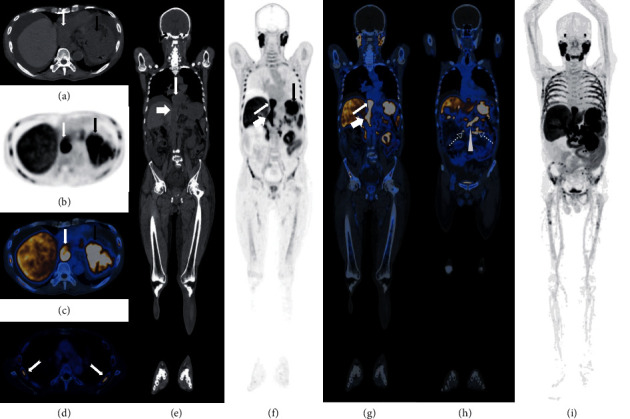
^18^F-PSMA PET/CT images of the same patient. (a–d) Transverse sections of CT, PET, and PET/CT fusion soft tissue window and PET/CT fusion bone window. (e–h) Coronal sections of CT, PET, and PET/CT fusion images, respectively. (i) PET MIP image. The long black arrow in (a–c) and (e–h) indicates gastric metastasis. The long white arrow in (a–c) and (e–g) indicates cancer thrombus in the right atrium. The short white arrow in (e-g) indicates cancer thrombus in the inferior vena cava. The white triangle in (h) indicates cancer thrombus in the splenic vein. The white dovetail arrows in (d) indicate multiple bone metastases. The white virtual arrows in (h) indicate abdominal lymph nodes.
